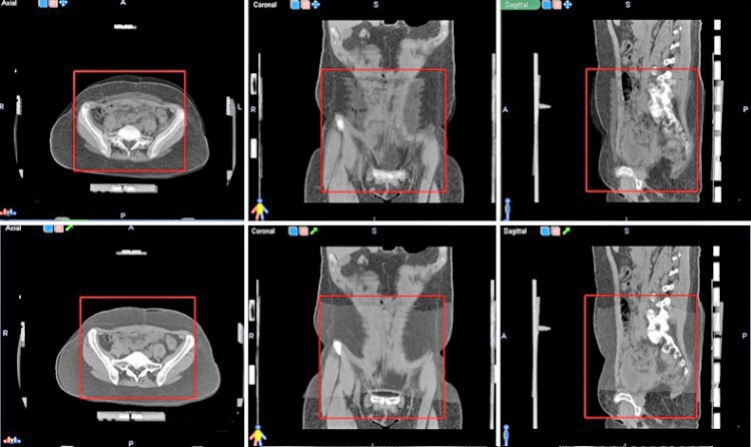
Definition of the region of interest (ROI) for DIR in Velocity AI: (first row) the planning CT and the original fraction CT are superimposed; (second row) a fusion of the planning CT and the deformed fraction CT. Only image information inside the red ROI is used to determine the transformation.
